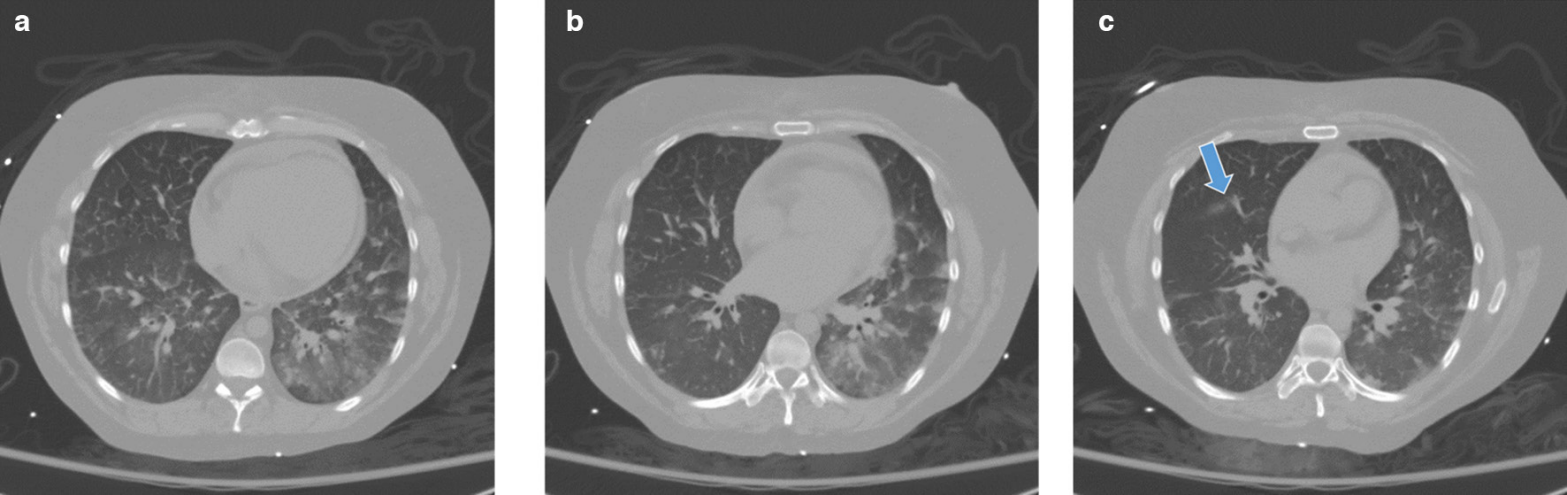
(A-C) Additional sequential imaging from the pre-contrast images taken during CT angiography on date of vascular injury. A. Axial pre-contrast imaging of the lungs near the base of the middle lobe on the date of vascular injury demonstrating no evidence of pulmonary hemorrhage involving the middle lobe prior to contrast media administration. B. Axial pre-contrast CT image a few slices more superior at the level of the proximal segmental artery branches of the middle lobe demonstrating no evidence of parenchymal hemorrhage involving the middle lobe. C. Axial pre-contrast CT imaging of the lungs more superior, now near the top of the middle lobe again demonstrating no evidence of pulmonary hemorrhage involving the middle lobe. Note the presence of the minor fissure (arrow), not to be confused for hemorrhage.
